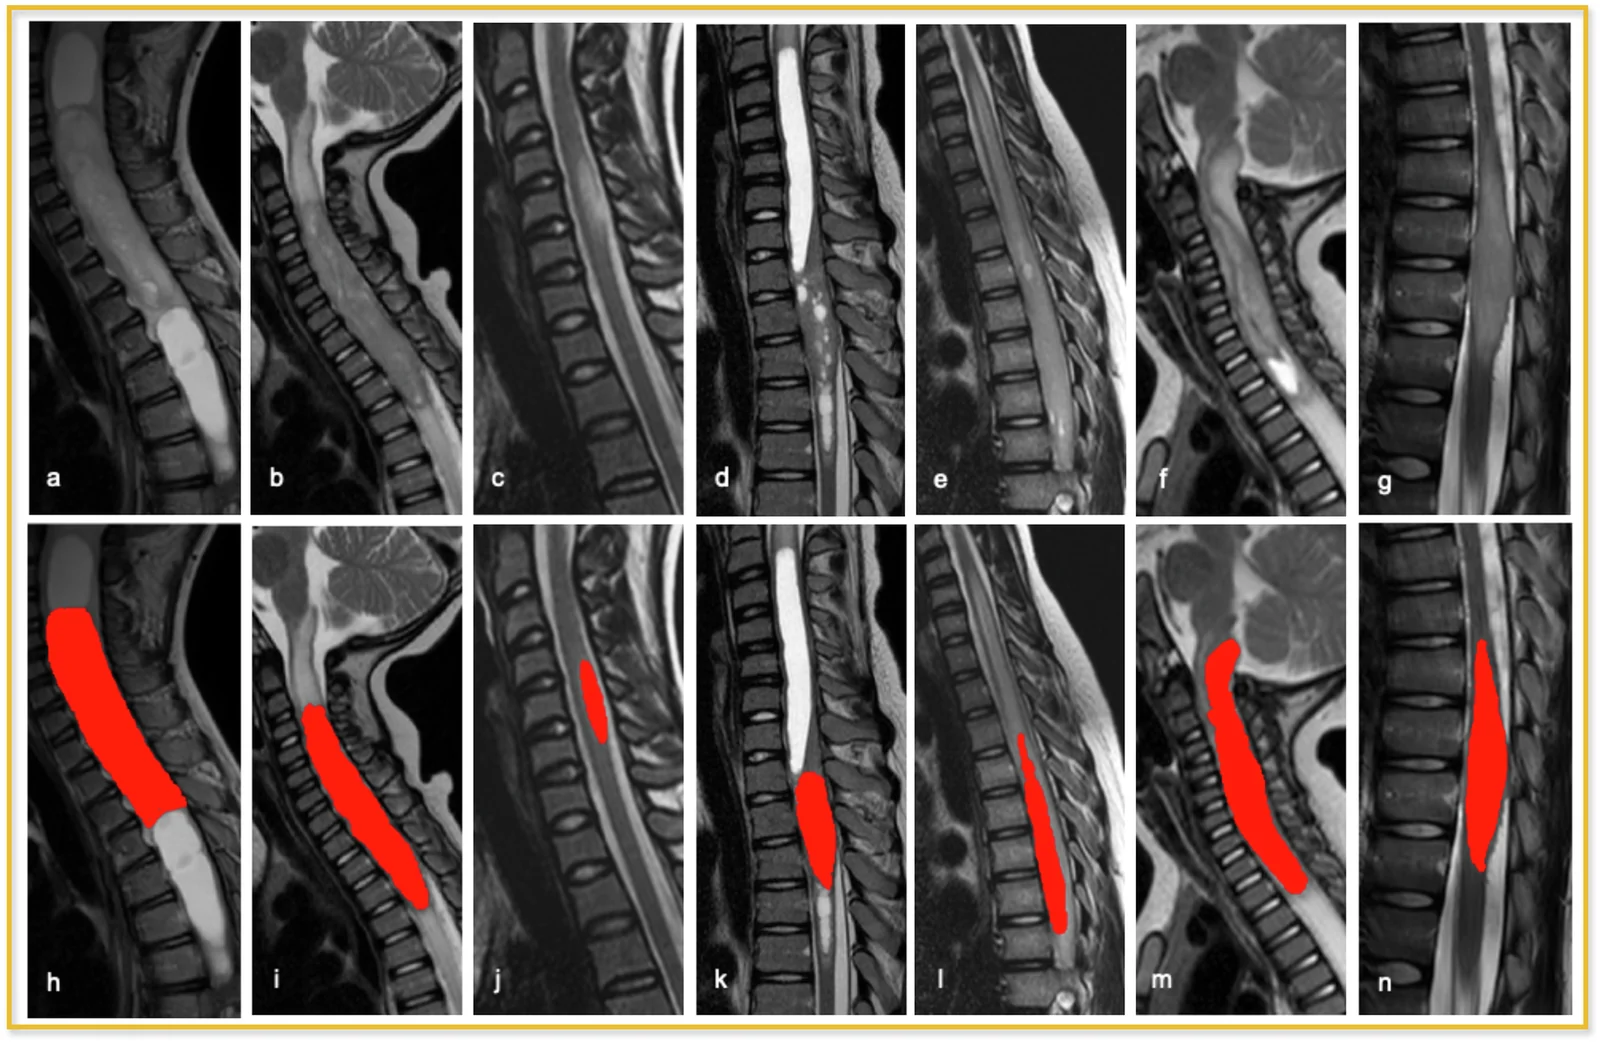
Conventional MRI features of selected IMSTs prevalent in pediatric patients. The figure includes sagittal T2-weighted images (a–g) next to manual segmentations performed using ITK-Snap (h–n). Specifically, the tumors featured are pilocytic astrocytoma (WHO Grade I) (a, h), ganglioglioma (WHO Grade I) (b, i), subependymoma (WHO Grade I) (c, j), ependymoma (WHO Grade II) (d, k), anaplastic astrocytoma (WHO Grade III) (e, l), glioblastoma (WHO Grade IV) (f, m), and diffuse midline glioma (WHO Grade IV) (g, n). Note the partially overlapping characteristics of these tumors across conventional MRI sequences. IMSTs, Intramedullary spinal tumors; MRI, Magnetic resonance imaging; WHO, World Health Organization
